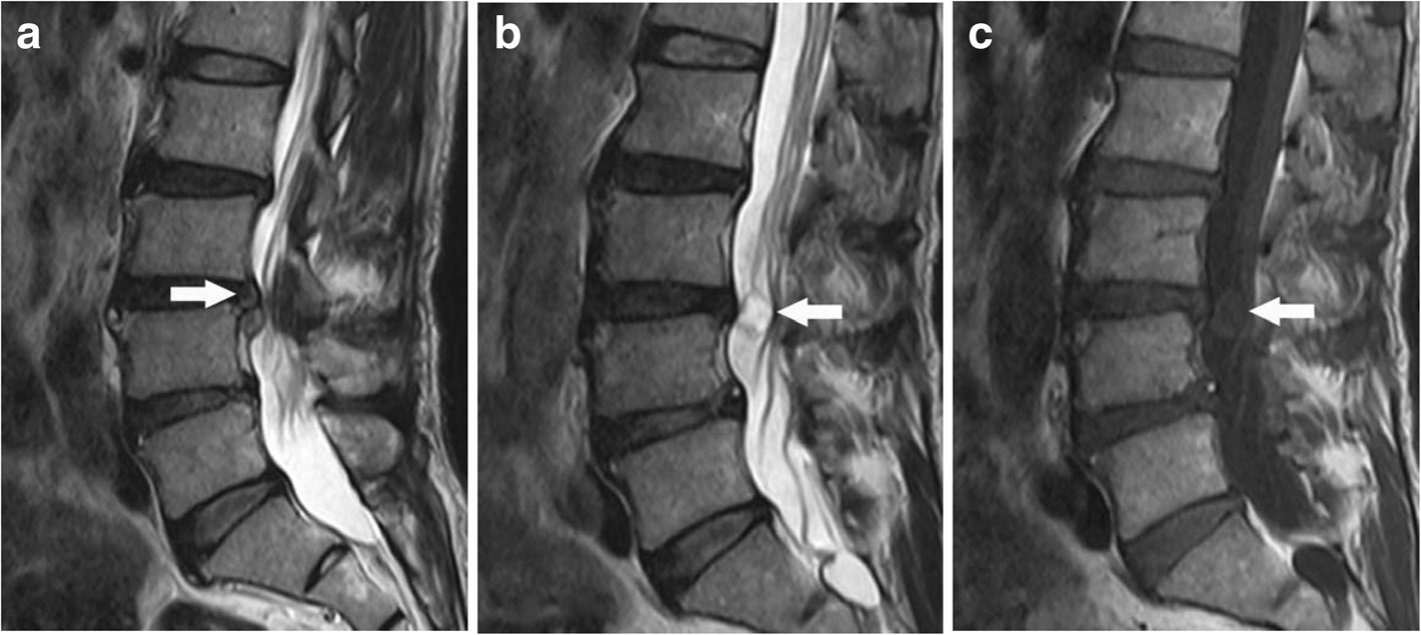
T2-weighted sagittal image (a) revealed L3/4 disc herniation which compressed the dural sac from the right side. Two slices away, there was a hyperintense intradural mass at the same level (b). T1-weighted sagittal image (c) demonstrated a hypointense mass behind the L3/4 intervertebral disc
